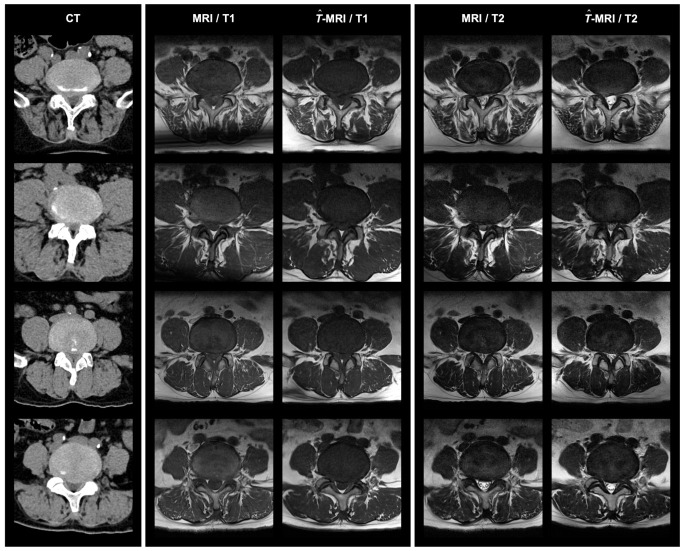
Representative axial slices comparing CT, MRI, and $\hat{T}$-MRI. Columns show CT, MRI/T1, $\hat{T}$-MRI/T1, MRI/T2, and $\hat{T}$-MRI/T2. Each row represents a different case at representative lumbar levels. $\hat{T}$-MRI is shown in the same spatial geometry as CT to support disc-related assessment.
